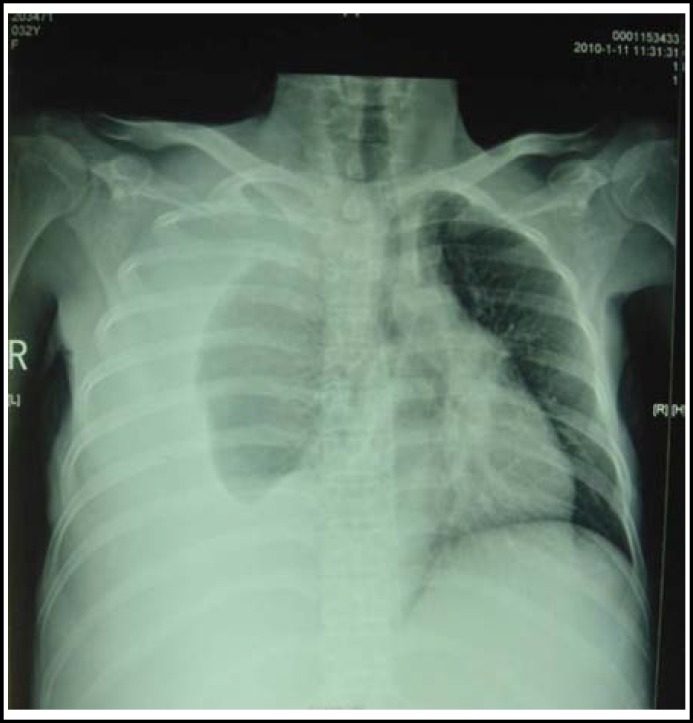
Chest X-rays examination showed a lot of right pleural effusion
